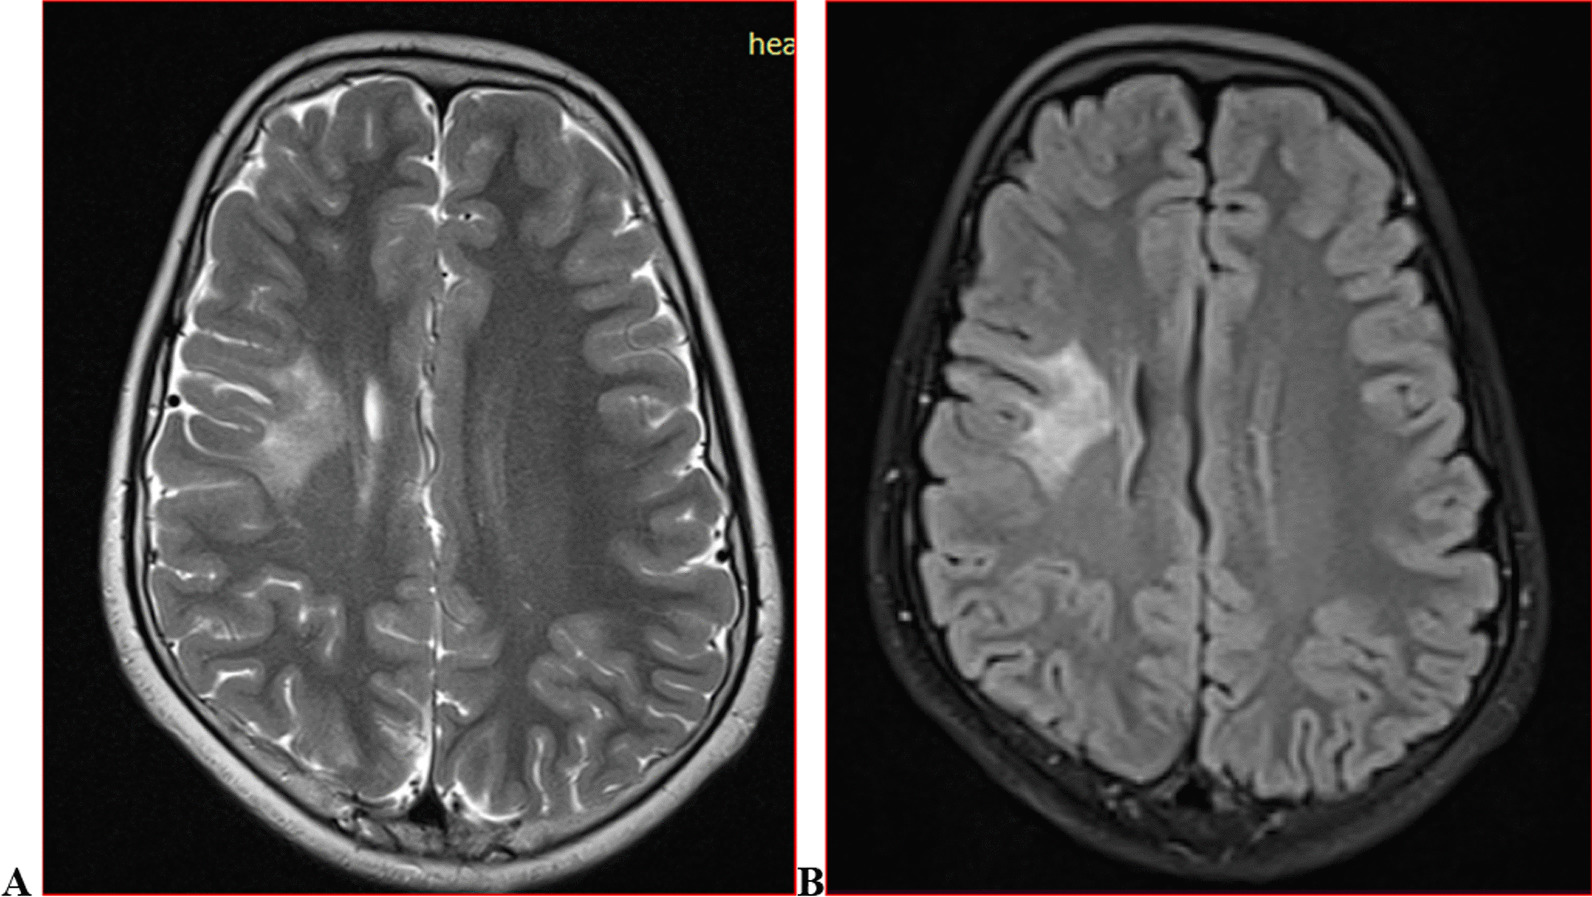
Twelve year old male with epilepsy brain axial MRI (T2/FLAIR) (A and B respectively) demonstarting subcortical white matter T2/FLAIR hyperintensity with a broad base towards the bottom of sulcus and tapering towards the lateral ventricle. The overlying gyrus appears dysplastic with local widening of the subarachnoid space. These findigs were highly sugestive of Focal Cortical Dysplasia
